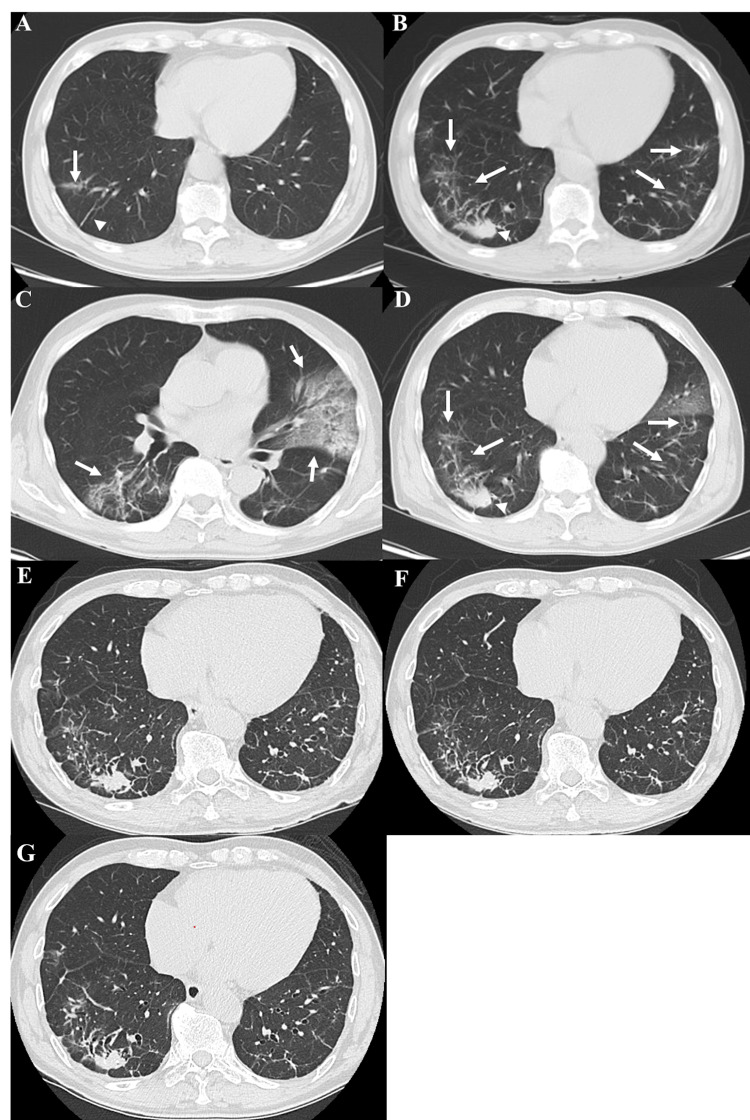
Chest CT images. (A) A CT scan obtained five years ago shows a slight GGO (arrow) and a linear shadow (arrowhead) in the right lower lobe. (B) CT scan performed one month before presentation shows GGOs in both lower lobes (arrows) of the lungs and a nodular shadow in the right lower lobe (arrowhead). (C, D) CT scan on admission showed a new GGO in the left upper lobe (C, arrow), while the previously existing GGOs and nodules in the lower lobes remained largely unchanged (D, arrows and arrowhead, respectively). (E) CT scan performed at the initiation of tafamidis meglumine therapy. (F) Follow-up CT scan after two months of treatment showing stability of the opacities in the right lower lobe. (G) Follow-up CT scan after one year of tafamidis therapy showing continued radiologic stability of the pulmonary opacities. GGO: ground-glass opacity
